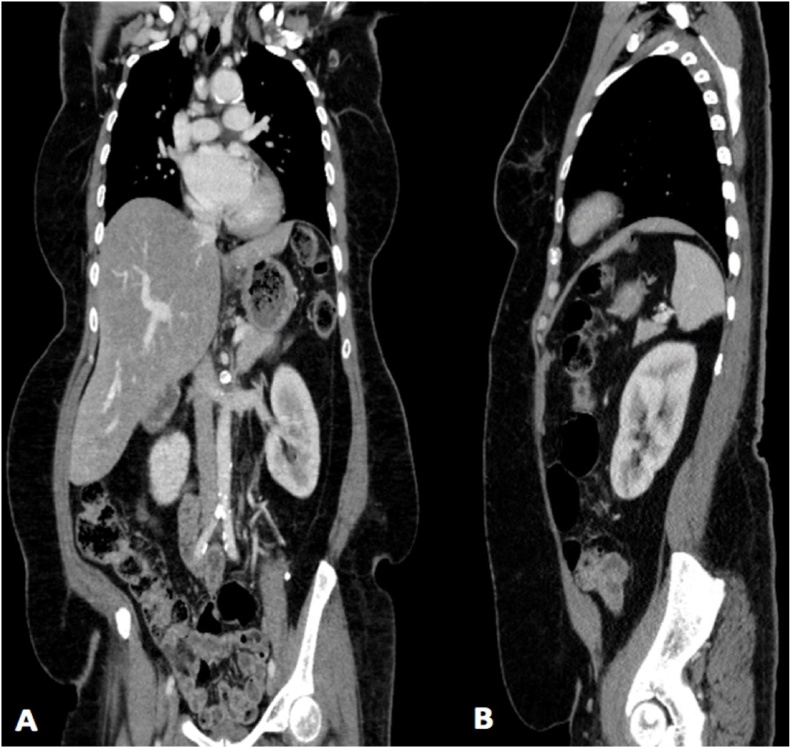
A: Coronal view of computed tomography scans of the chest and abdomen after 7 years from first surgery showing no local recurrence and no new lesions. B: Sagittal view of the same image.
